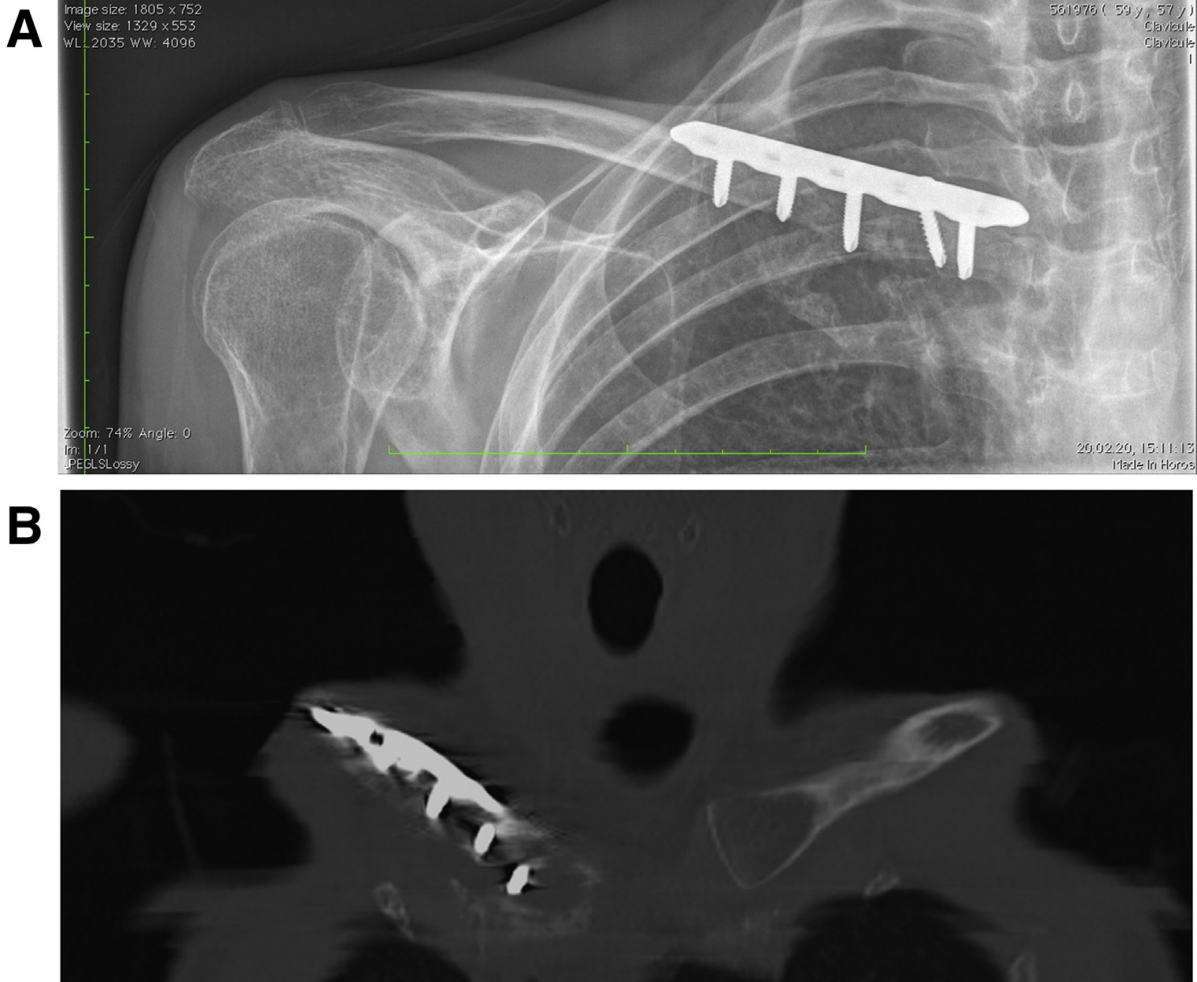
Repeat AP radiograph of the right clavicula (A) at eight weeks and CT (coronal view) of the right clavicula (B) at 1 year after operation show bony consolidation of the bone graft with restoration of the clavicle length. AP, anteroposterior; CT, computed tomography.
